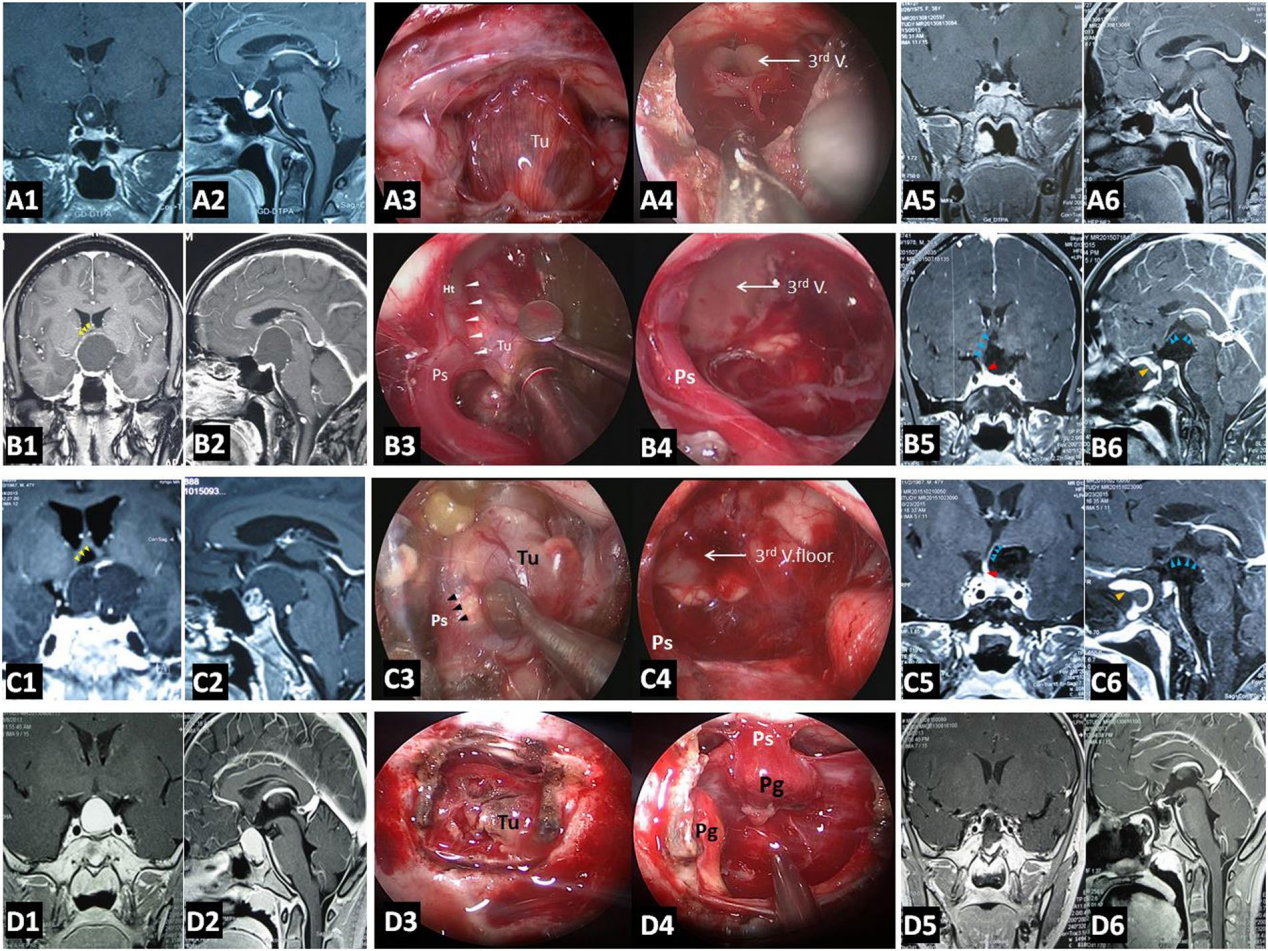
Pre- and post-operative images and intraoperative findings of each subtype of CPs via endoscopic endonasal approach. (A1-6) A case of Central type CP. (A1-2) Coronal and sagittal postcontrast T1-weighted MR images showing a Central type CP which is located in the midline. (A3-4) Intraoperative photographs showing that tumor grew within and along the stalk, and the characteristic structure of stalk could be clearly identified on the surface of tumor (A3). Bilateral hypothalamus damage limited to tuber cinereum area could be seen after tumor removal (A4). (A5-6) Coronal and sagittal postcontrast T1-weighted MR images obtained after GTR. The pituitary gland was preserved and intact, but the stalk was not preserved. (B1-6) A case of Hypothalamic stalk CP. (B1-2) Coronal and sagittal postcontrast T1-weighted MR images showing a suprasellar CP located slightly toward to the left side and grows into the third ventricle, with the anterior third ventricle shifted to the right side (yellow arrowheads in B1). (B3-4) Intraoperative photographs showing that tumor originated from the junction of the hypothalamus and the stalk (white arrowheads in B3), the stalk was pushed to the right side. After tumor removal, a defect at the left side of the third ventricle floor could be found, and the remnant of stalk was pushed to the right side and connected to the right side of the third ventricle floor, with a relative normal hypothalamus at the right side (B4). (B5-6) Coronal and sagittal postcontrast T1-weighted MR images showing total tumor removal achieved. The stalk was preserved and pushed to the right side (red arrowhead in B5), the right hypothalamus was intact (blue arrowheads in B5-6). Note the vascularized nasoseptal flap at the posterior aspect of the sphenoid sinus on the sagittal image (orange arrowhead in B6). (C1-6) A case of Suprasellar stalk CP. (C1-2) Coronal and sagittal postcontrast T1-weighted MR images showing a suprasellar CP located toward to the left side, with the anterior third ventricle shifted to the right side (yellow arrowheads in C1). (C3-4) Intraoperative photographs showing that tumor derived from suprasellar segment, low portion of the stalk (black arrowheads in C3). After tumor removal, the third ventricle floor was intact, with no hypothalamus damage, and the remanent of stalk was pushed to the right side (C4). (C5-6) Coronal and sagittal postcontrast T1-weighted MR images showing total tumor removal achieved. The stalk was preserved and pushed to the right side (red arrowhead in C5), and the hypothalamus was intact (blue arrowheads in C5-6). Note the vascularized nasoseptal flap at the posterior aspect of the sphenoid sinus on the sagittal image (orange arrowhead in C6). (D1-6) A case of Intrasellar stalk CP. (D1-2) Coronal and sagittal postcontrast T1-weighted MR images showing a typical intrasellar CP. (D3-4) Intraoperative photographs showing that the solid tumor located in the sellar region. Residual pituitary gland and stalk can be seen after tumor removal (D4). (D5-6) Coronal and sagittal postcontrast T1-weighted MR images showing total tumor removal achieved. Ht, hypothalamus; Tu, tumor; Pg, pituitary gland; Ps, pituitary stalk. 3rd V., the third ventricle.
